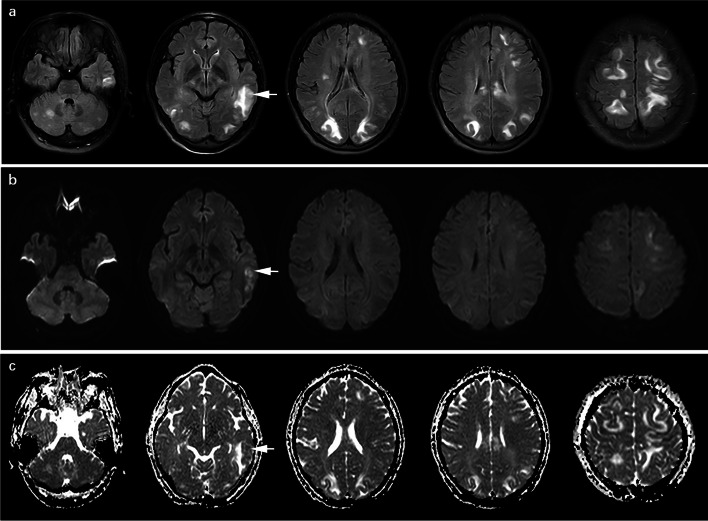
Cranial MRI of a 31-year-old woman with eclampsia with PRES: a axial T2W FLAIR-SPIR, b axial DWI with b value of 1000 s/mm^2^, c axial ADC map. SBE was evaluated according to the distribution and the degree of abnormal edema signal, which presented as heterogeneous hyperintense signal (arrow) on T2W FLAIR-SPIR (a). Large area fusions of abnormal edema signals were shown in corpus callosum, bilateral occipital, parietal, frontal, and temporal lobes; and multiple flecks, patches or nodules of abnormal edema signals without fusion were seen in the cerebellum and right basal ganglia. The SBE of typical regions, atypical regions, and all regions in this patient were 12, 19, and 31, respectively. Some of the abnormal edema signals in bilateral frontal lobes, left parietal and temporal lobes presented as hyperintense signals (arrow) on DWI (b), with the lesion in left temporal lobe locally appearing as hypointense signal (arrow) on ADC map (c)
